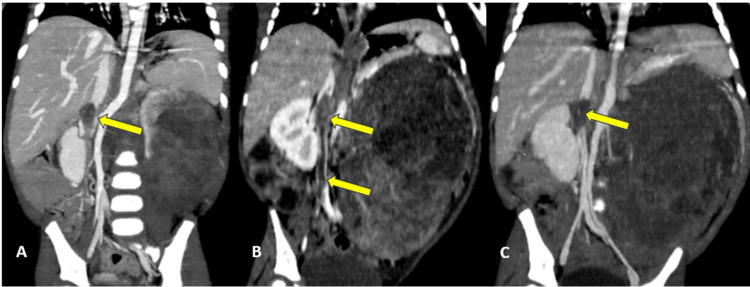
Coronal reconstructions of the initial abdominopelvic CT scan (A), first follow-up (B), and second follow-up (C) images showing the evolution of the intravascular thrombus within the IVC (yellow arrow). IVC, inferior vena cava
